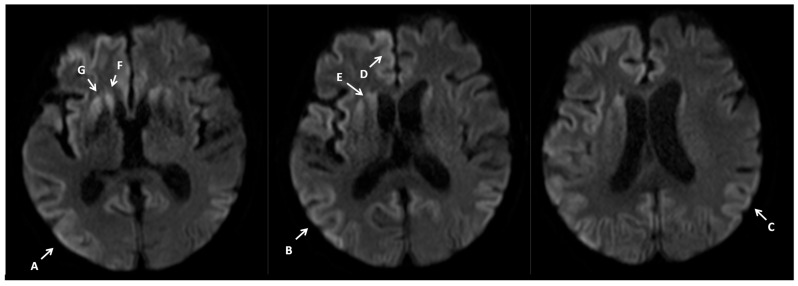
Contrast MRI imaging 10 June 2024. Imaging performed on a 3T scanner. Brain MRI shows diffuse increased signal on diffusion-weighted imaging (DWI) within the cerebral cortsex-cortical ribbon sign (A–D), caudate nuclei (E,F), and putamen (G), with changes more pronounced on the right side. Imaging burdened with movement artifacts.
